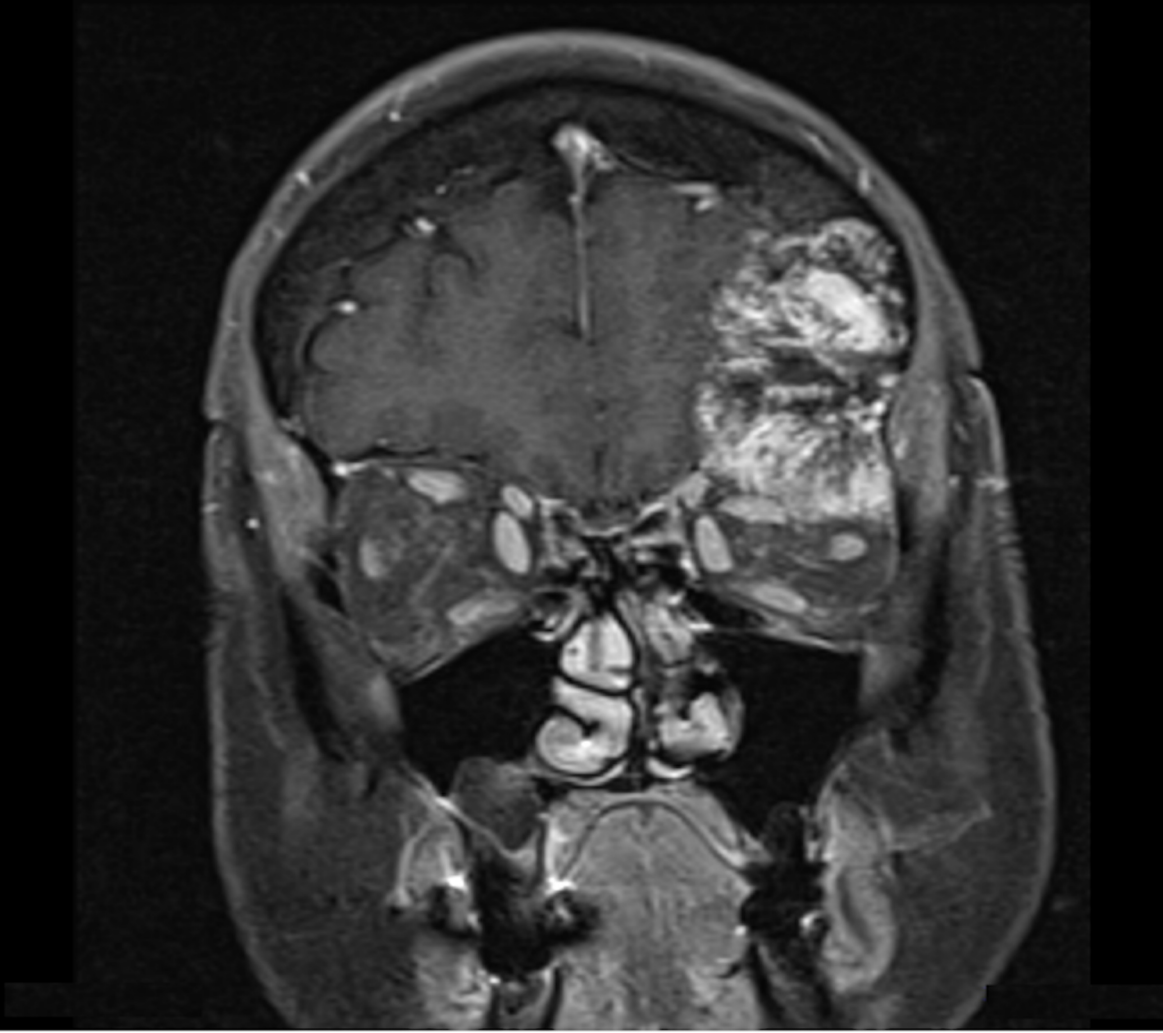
Preoperative coronal T1 contrast-enhanced magnetic resonance imaging
